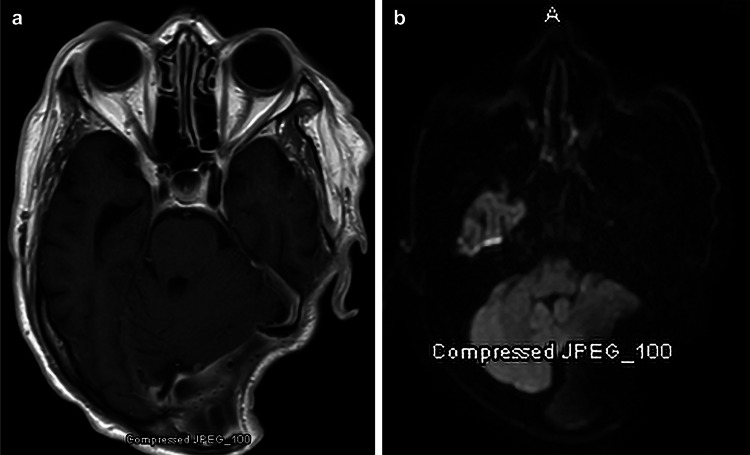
Six-month postoperative axial magnetic resonance imaging. (a) No signs of infection and near-complete tumor removal shown on T1-weighted image with gadolinium and (b) diffusion-weighted image. *Used with permission from Barrow Neurological Institute, Phoenix, Arizona.*
